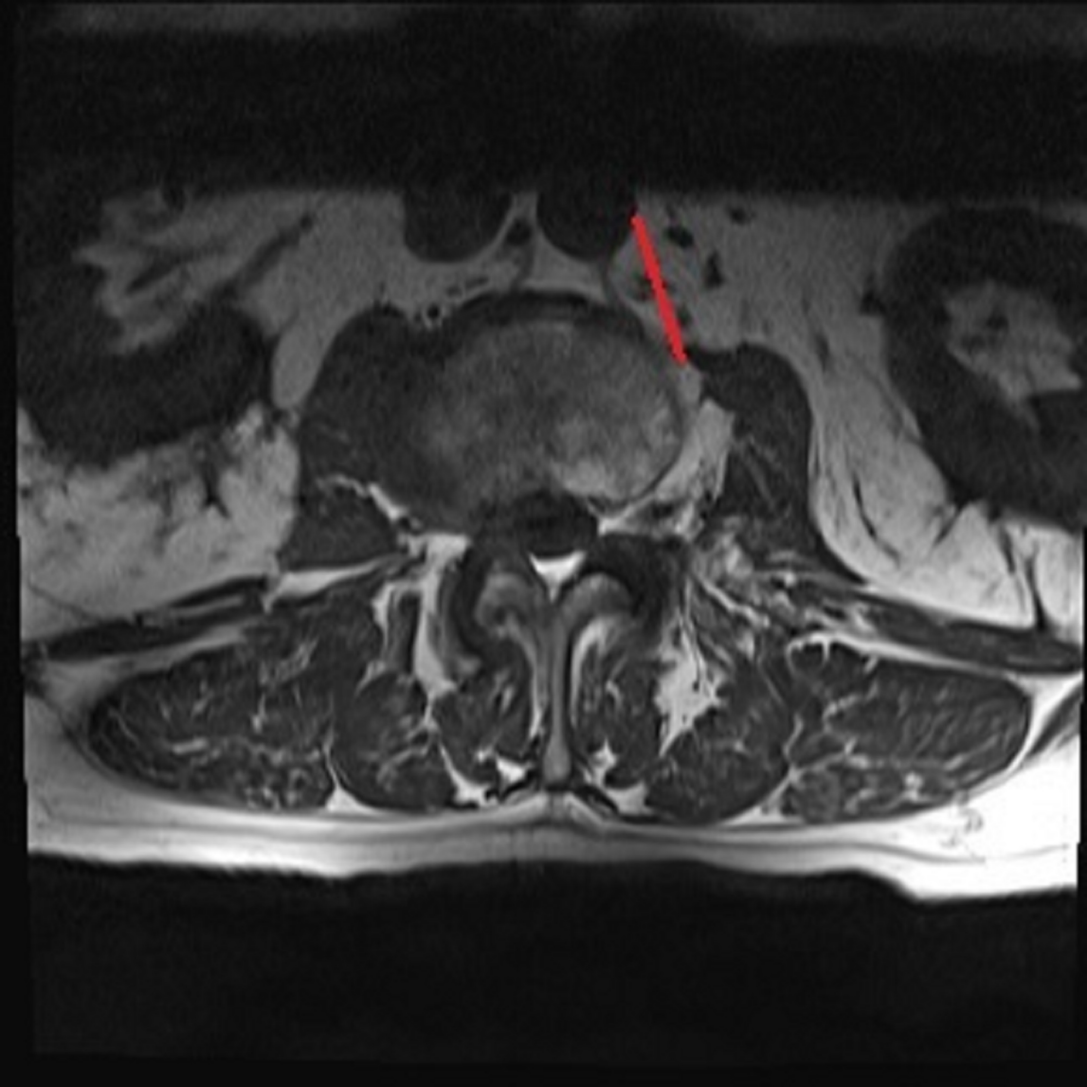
Preoperative corridor measurement using axial MRI T1 weighted image at the L2-L3 disc space as indicated by the red line MRI: magnetic resonance imaging
